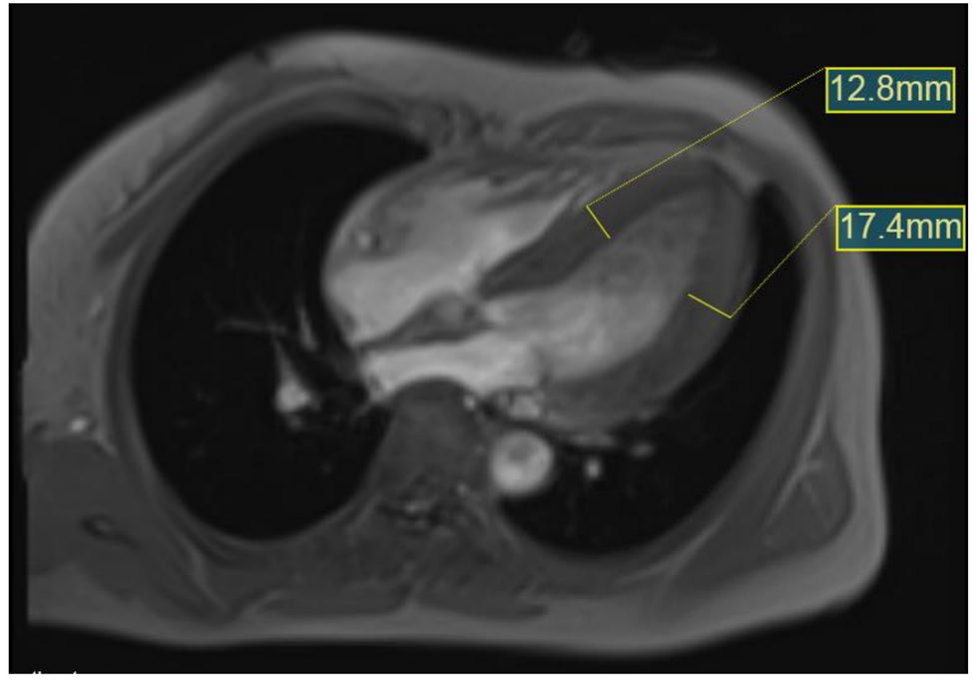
Cardiac magnetic resonance imaging results. The left ventricular septum and the left ventricular lateral wall in end-diastole are significantly thickened, with a maximum thickness of 17.4 mm. No significant enlargement of the left or right atrioventricular chambers and no expansion or stenosis of the pulmonary artery are observed
